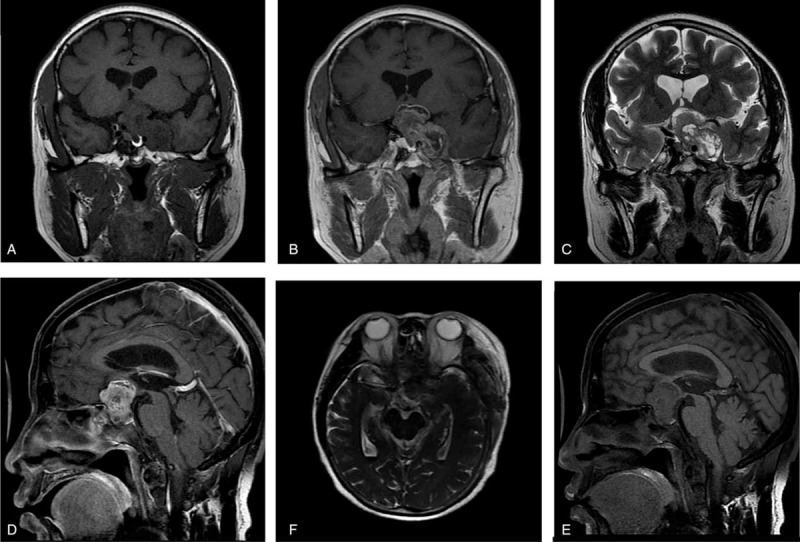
MRI showed an abnormal sellar region: a mixed-signal lesion with suprasellar, left parasellar, and sellar floor invasiveness. (A) coronal T1WI; (B) coronal enhanced-T1WI; (C) coronal T2WI; (D) sagittal T1WI; (E) sagittal enhanced-T1WI; and (F) axial T2WI.
